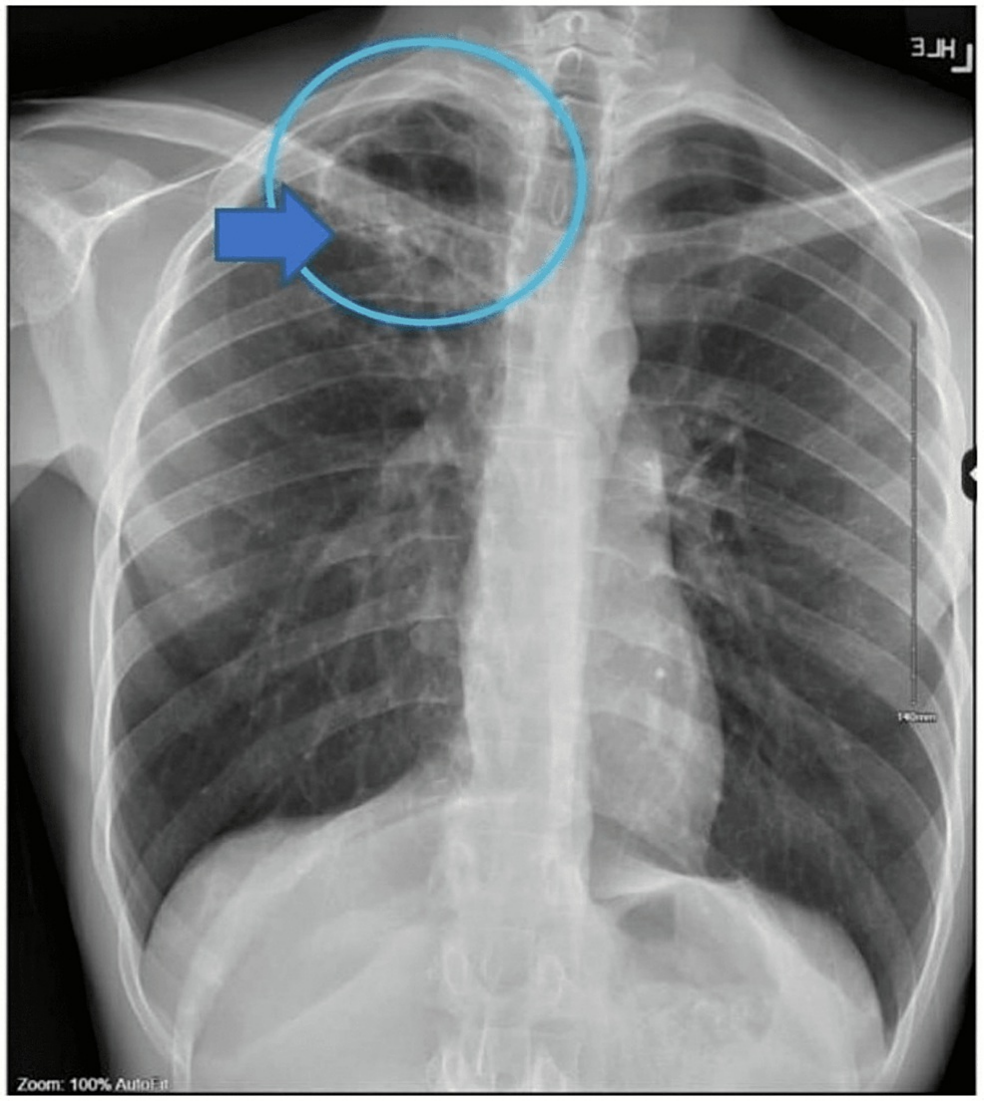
Chest X-ray showing findings of poorly defined infiltrates with a cavitary lesion in the right upper lobe (arrow)
